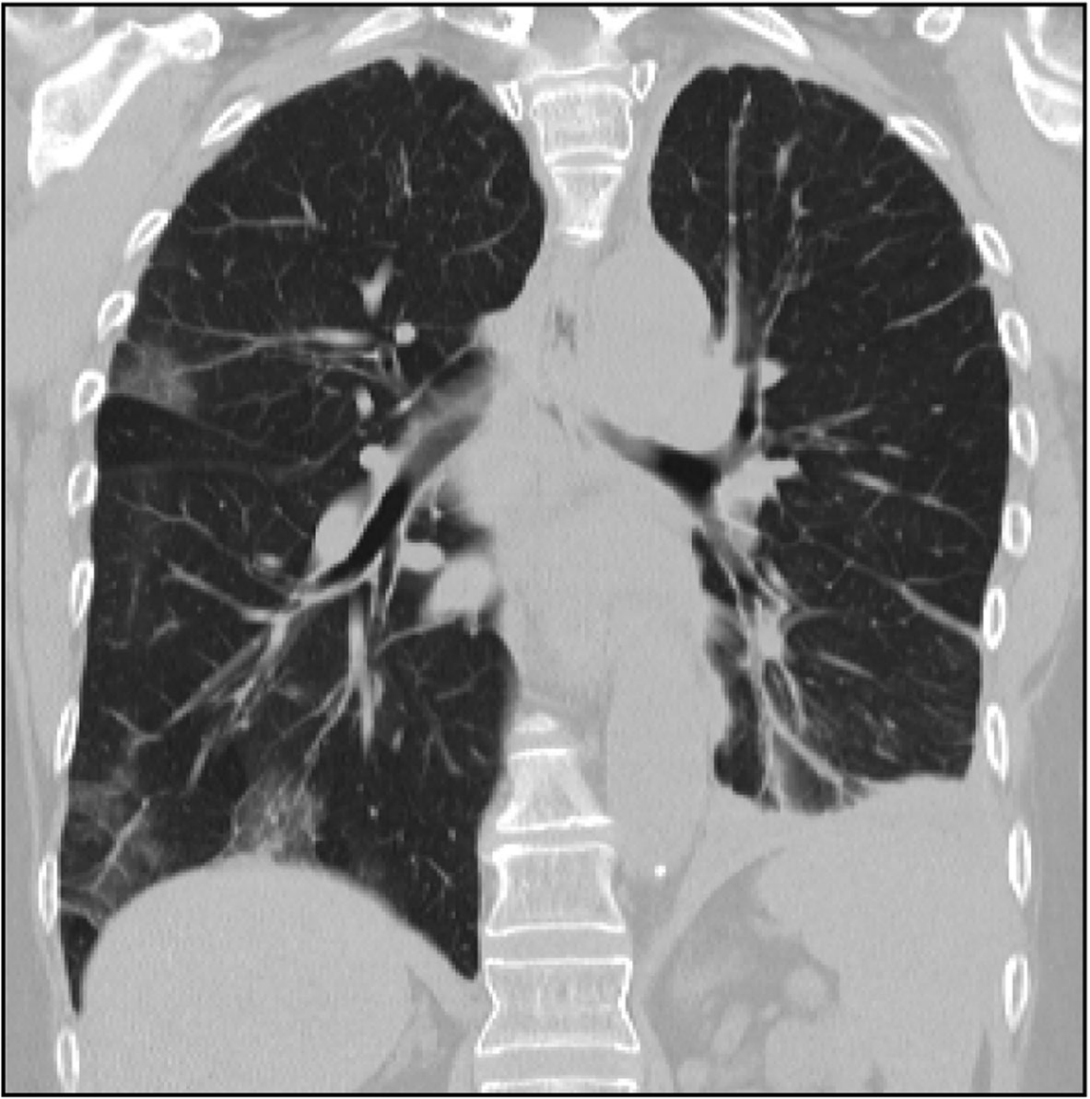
Female patient 70 years old with COVID-19. CT coronal image shows peripheral ground glass opacities with vascular thickening in the right lung. Note the left mild pleural effusion
